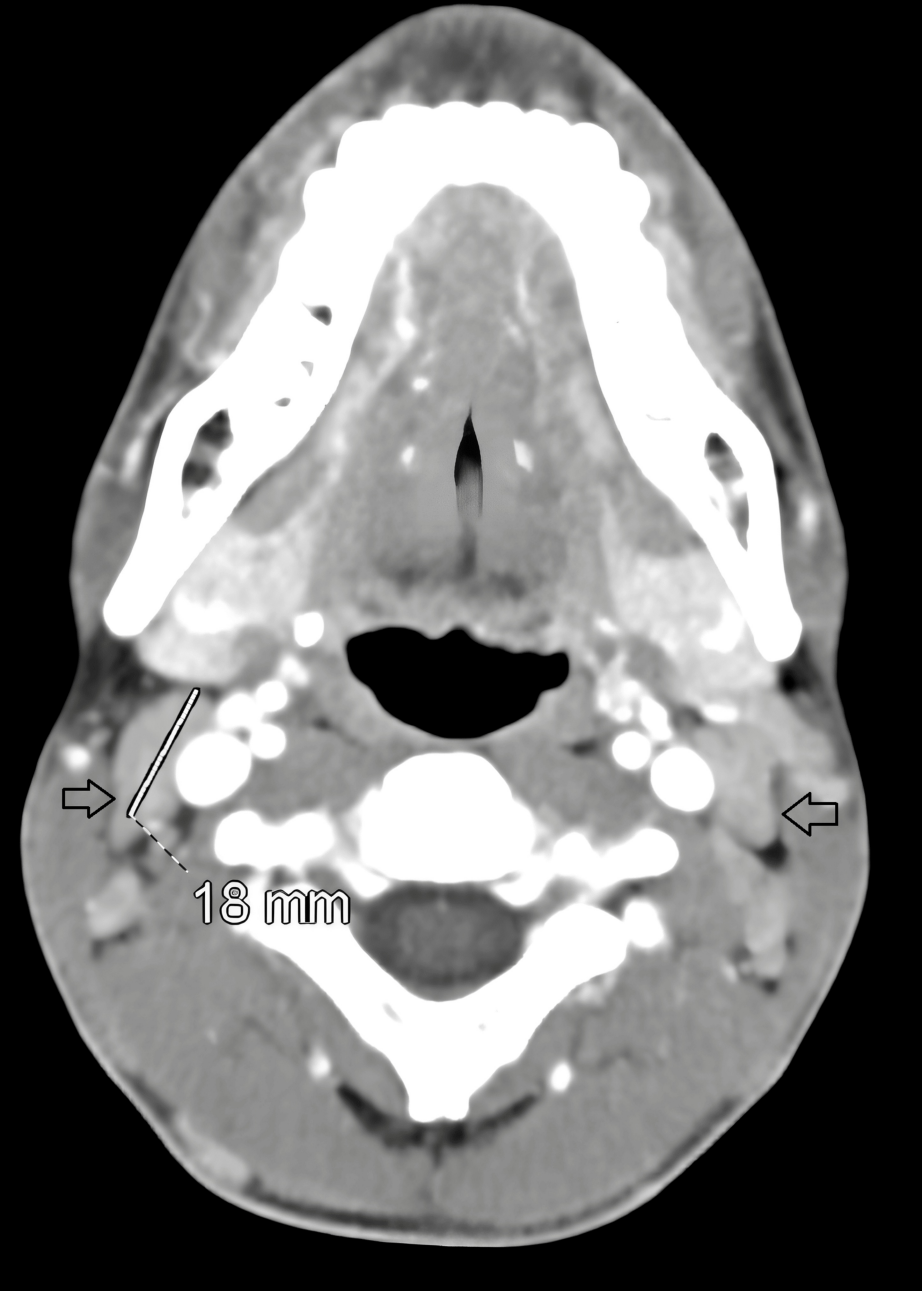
Neck and chest CT revealed multiple enlarged lymph nodes in the bilateral cervical region (black arrows), largest one in the right cervical region measuring up to 1.8 cm (white line) CT: computerized tomography
